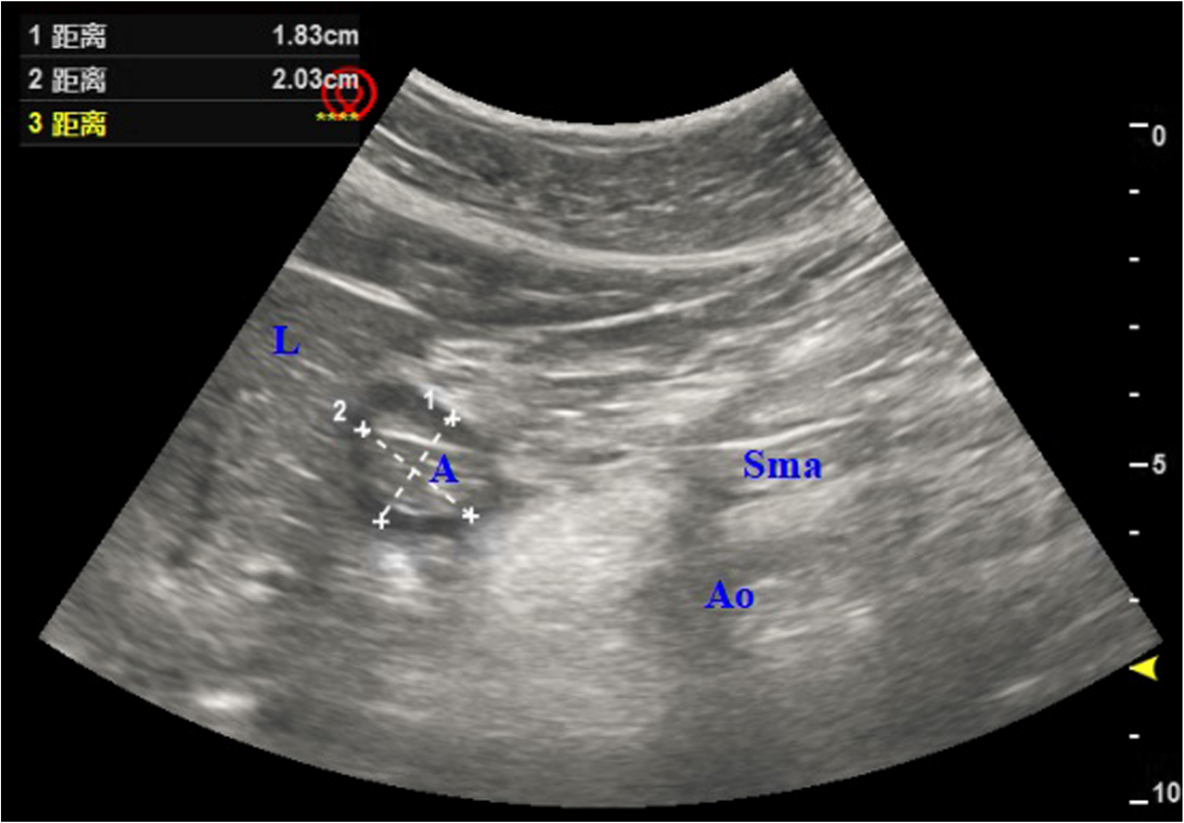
Two-Diameter Method was carried out to measure antral CSA by ultrasound before and after SJOV in the WNJ SJOV group. GA = Gastric antrum, Ao = aorta, Sma = superior mesenteric artery, L = liver. The antero-posterior (AP) antral diameter was expressed as dotted line 1,The cranio-caudal (CC) antral diameter was represented by dotted line 2,and π was 3.1416 during CSA = (AP × CC × π)/4
